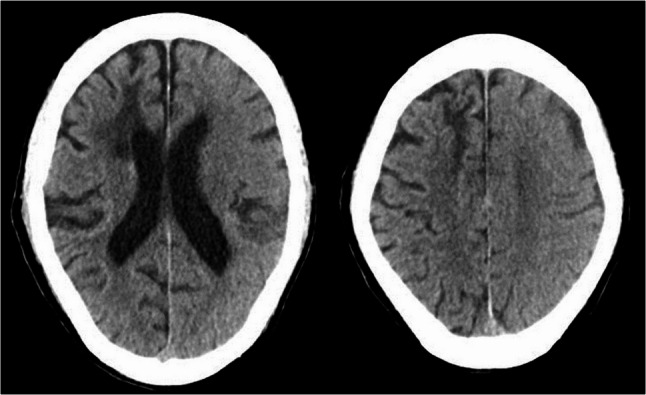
Non-contrast CT on POD2. CT showing partial resolution of edema and attenuation. CT: computed tomography. POD: postoperative day
